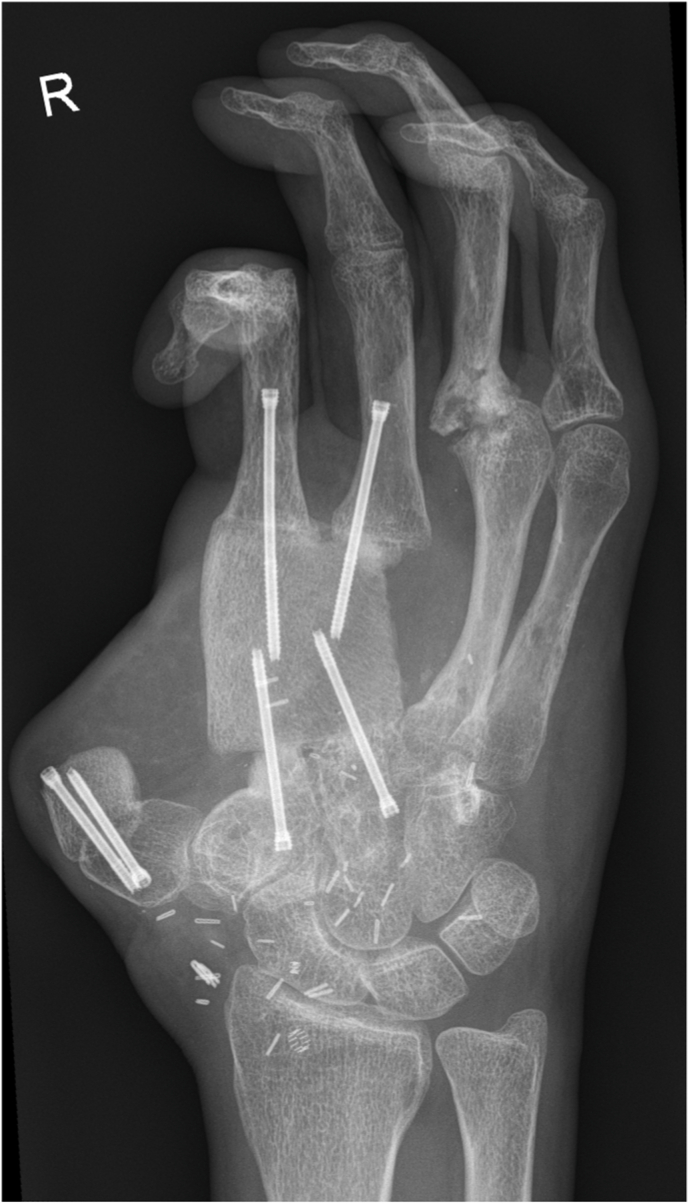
Early postoperative radiograph after reconstruction with an osteocutaneous SCIP flap, replacing the second and third metacarpal bones.
